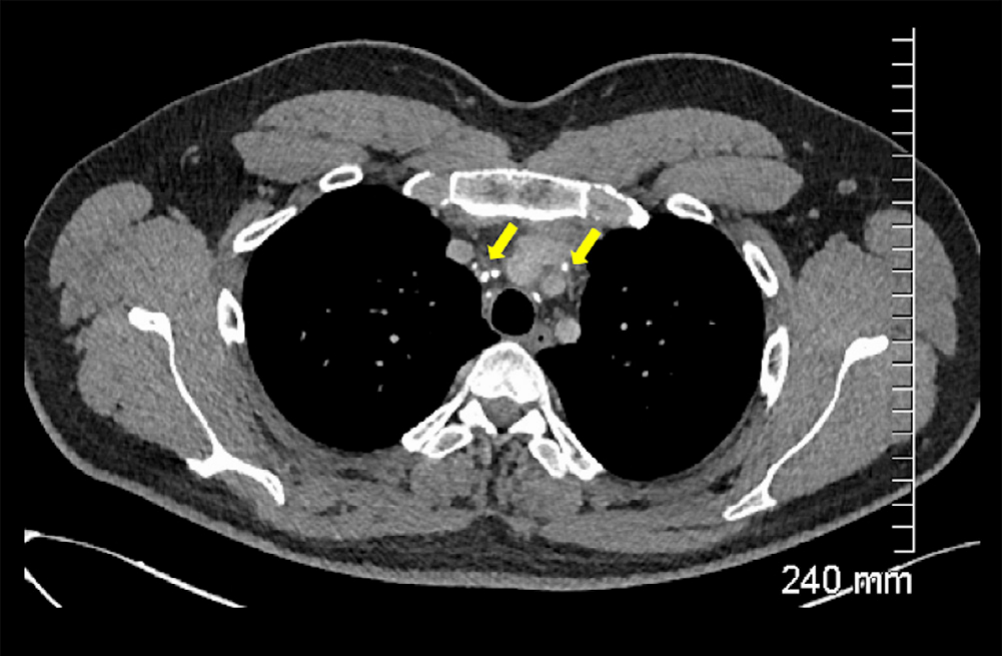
Axial contrast enhanced CT of the thorax demonstrating calcified small mediastinal lymph nodes (arrows).
